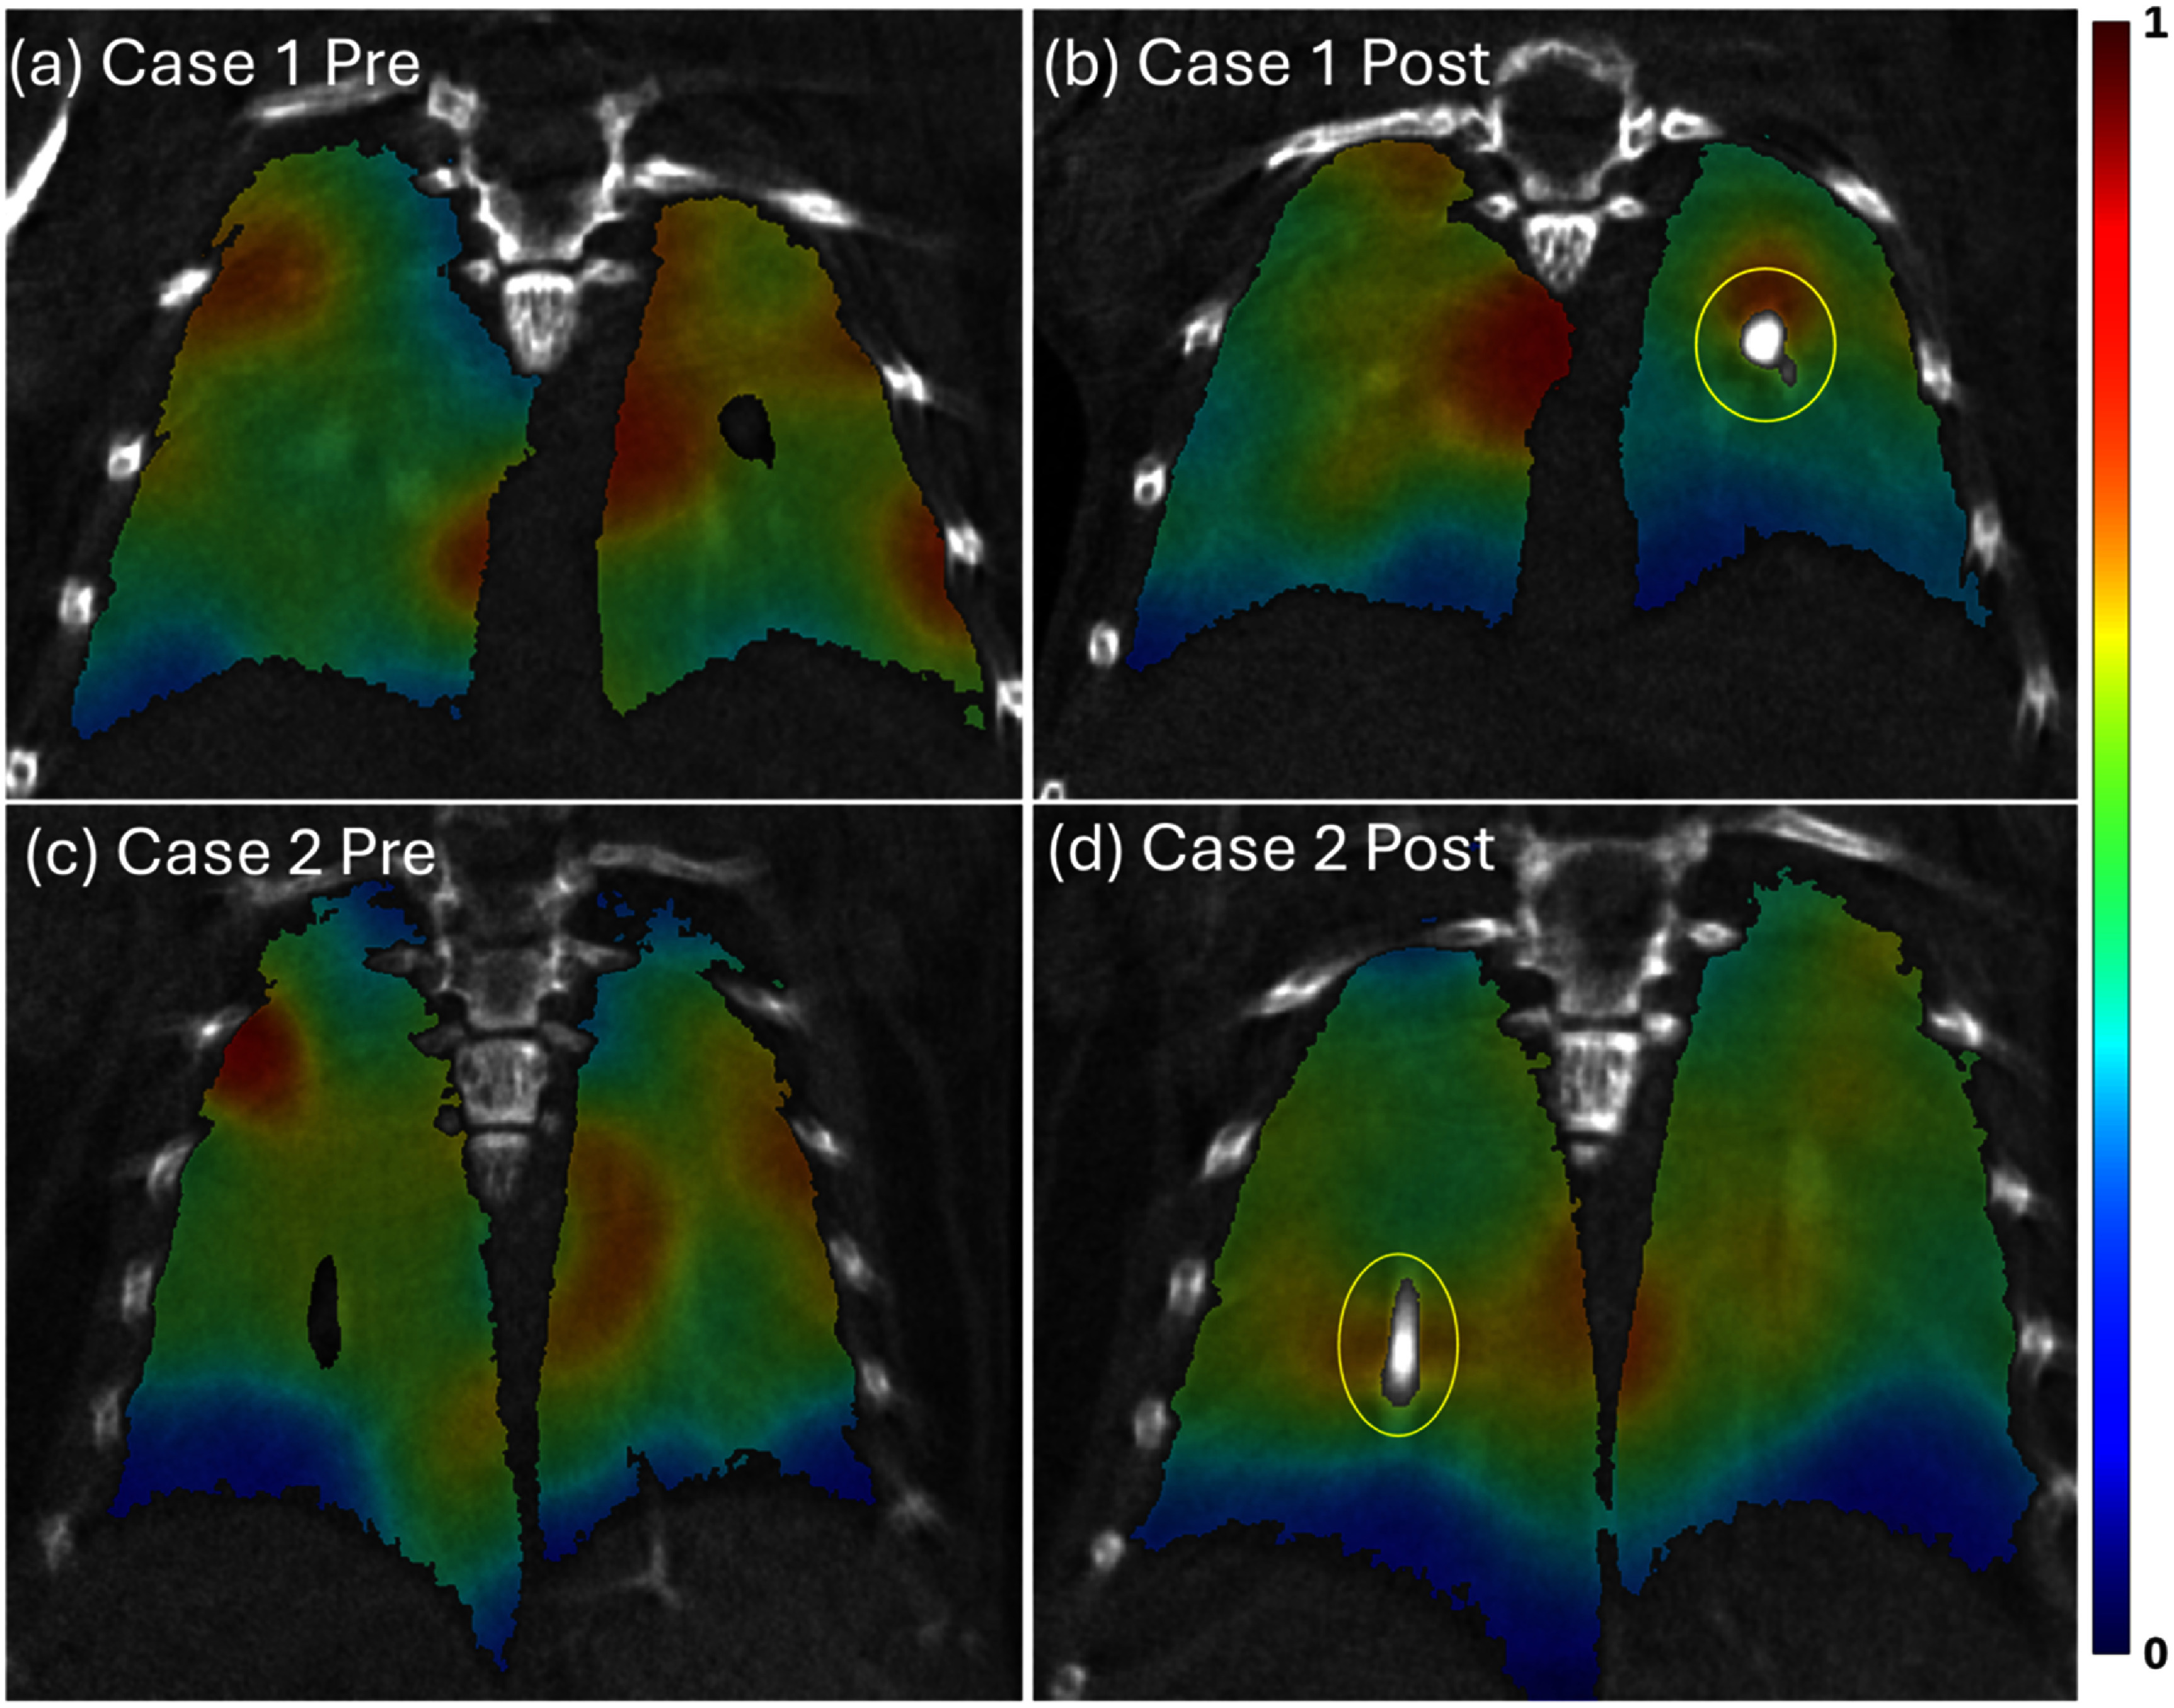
Pre- and post-embolization CT-P maps for Case 1 (a) and (b) and Case 2 (c) and (d), shown as representative coronal slices. Perfusion defects are visible in the post-embolization maps (b) and (d). Yellow circles indicate embolus locations, and the corresponding regions in the pre-embolization maps were excluded for fair comparison. The intensities were normalized within each case across the pre- and post-embolization images to enable direct visual comparison. The colorbar represents normalized CT-P values, where red indicates high perfusion and blue indicates low perfusion.
